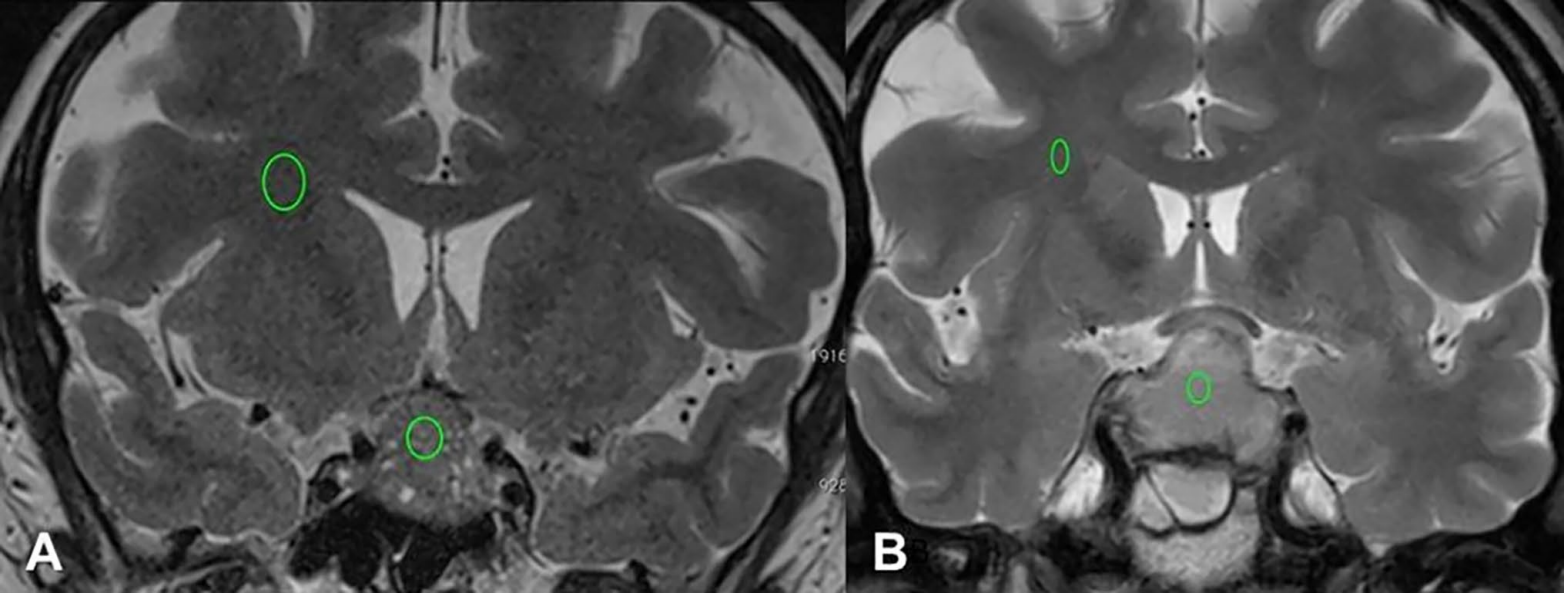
The role of T2 signal intensity-to-white matter ratio in corticotroph tumors. A A 52-year-old female with a diagnosis of densely granulated corticotroph tumor showed a pituitary tumor that occupied the sella and suprasellar cistern, with compression of the optic chiasm. The tumor appeared isointense to gray matter on T2-weighted imaging. The T2 signal-to-white matter ratio of the lesion was reduced to 0.026. B A 34-year-old female patient with a diagnosis of sparsely granulated corticotroph tumor was found to have a pituitary tumor on the sella and suprasellar cistern, with compression on the optic chiasm. The tumor appeared hyperintense to gray matter on T2-weighted imaging. The T2 signal-to-white matter ratio of the lesion was increased to 0.052
